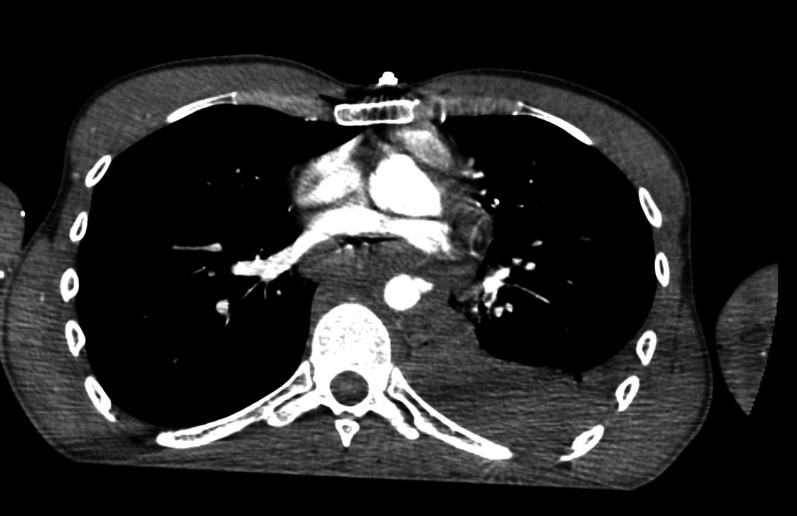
Axial angio-CT image showing contrast leakage from the thoracic aorta.
